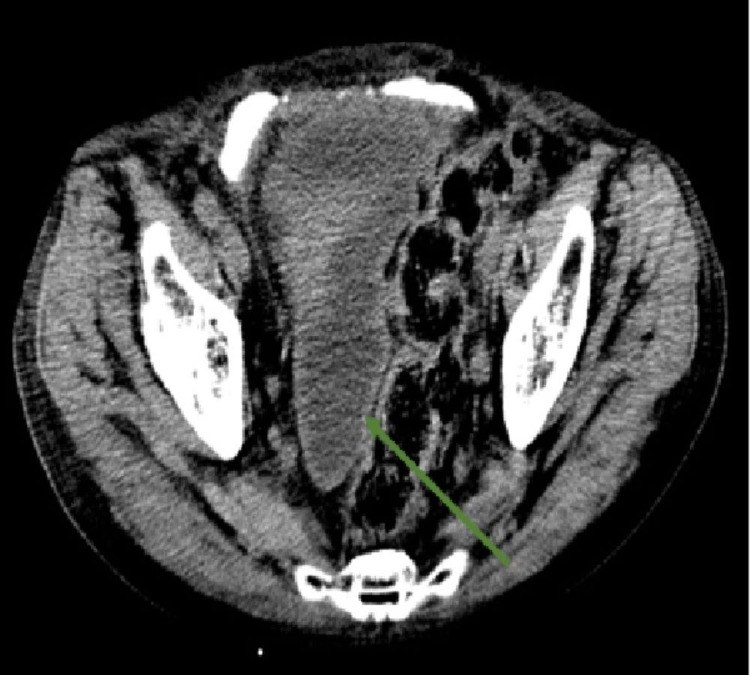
Computed tomography on day seven Contrast computed tomography revealed pelvic abscess around bladder (arrow).
